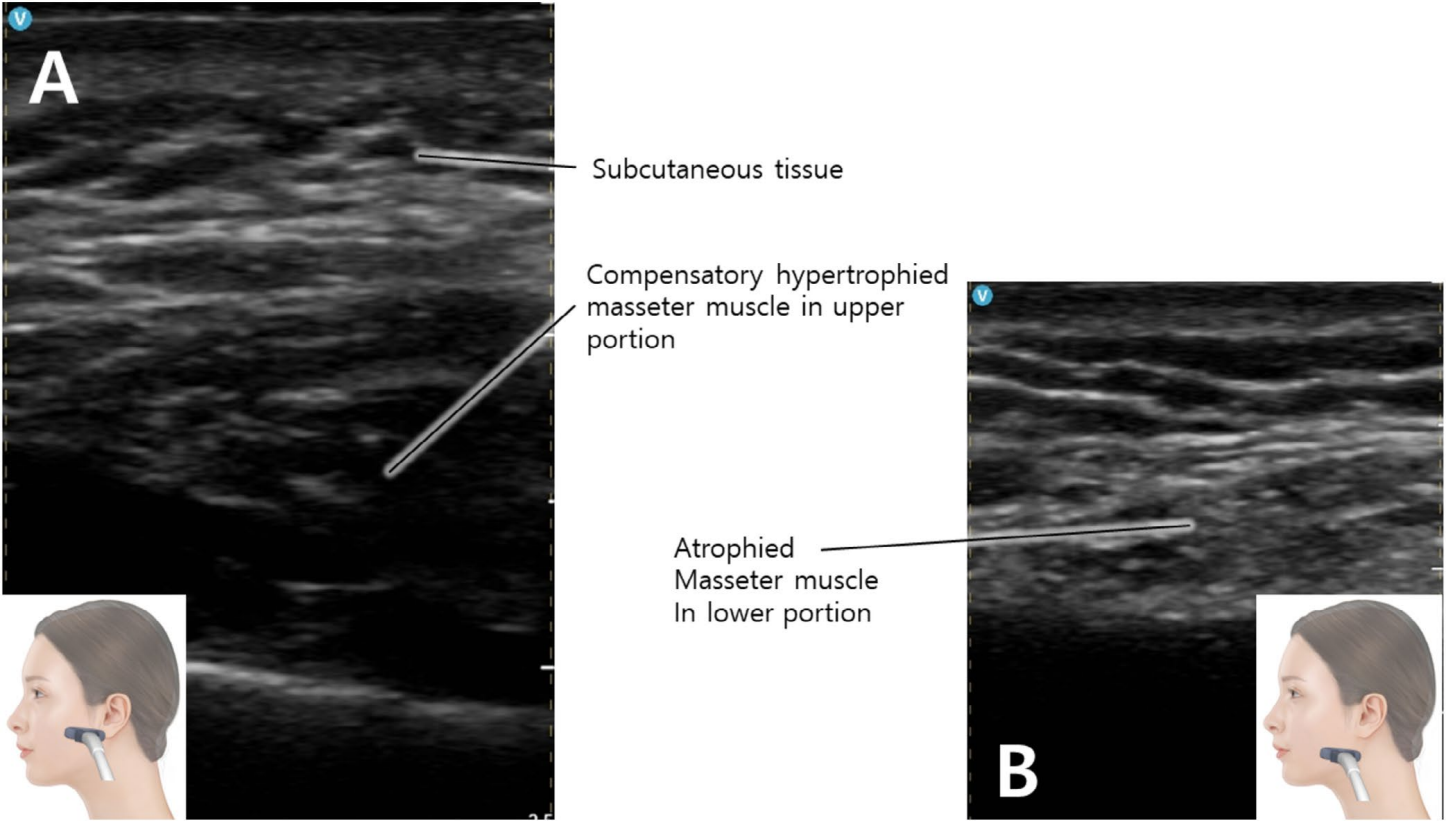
Ultrasound image of the patient's masseter muscle before additional treatment. The image shows a significant difference in thickness between the upper and lower portions of the muscle, with the upper section exhibiting increased bulk with a hypoechoic image at the upper portion (A). This hypertrophy was identified as a compensatory response to repeated botulinum neurotoxin injections in the lower mid‐part of the masseter. The atrophied masseter muscle in the lower portion is observed as a fibrotic hyperechoic image (B).
